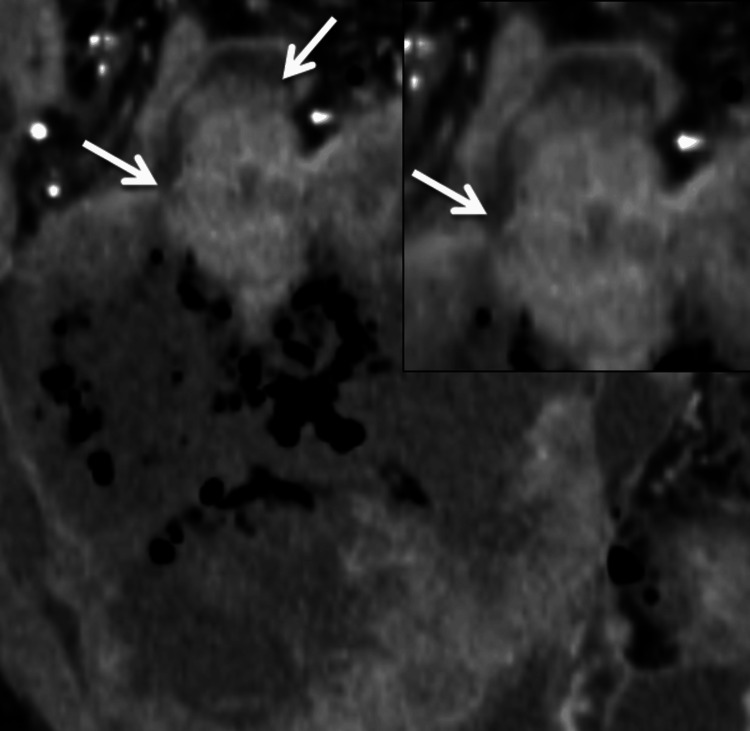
Coronal CECT image in venous phase demonstrating communication between the exophytic mass and adjacent small-bowel loops (white arrows) CECT: contrast-enhanced computed tomography
